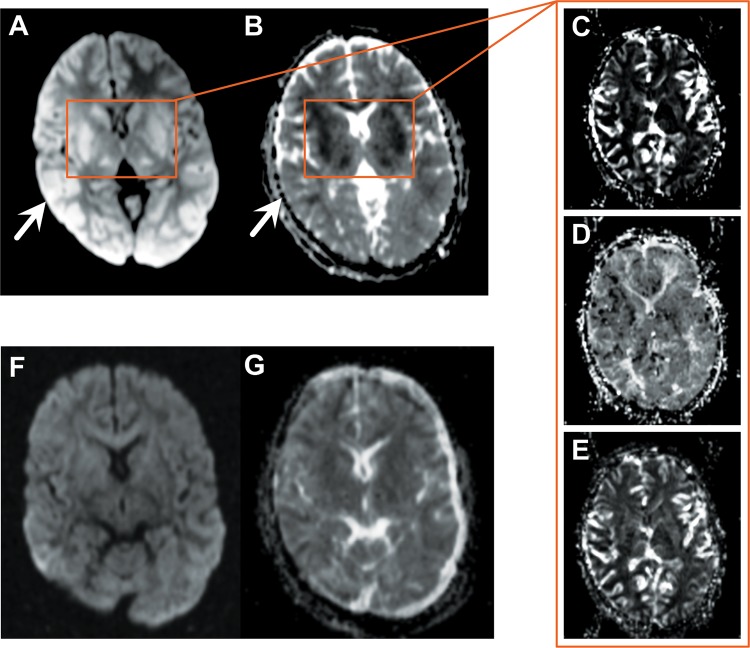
Case ID60. (A to E) Increased DWI signal in the posterior cortex (A, white arrow) with no mismatch in corresponding ADC image (B, white arrow). DWI and ADC mismatch in the basal ganglia (A and B, orange boxes) due to vascular engorgement demonstrated by an increased CBV (C), slightly delayed MTT (D), and nearly normal CBF (E). (F, G) Interval DWI image (F) and corresponding ADC map (G) demonstrating a complete resolution of the lesions 48 to 72 h postadmission and treatment.
